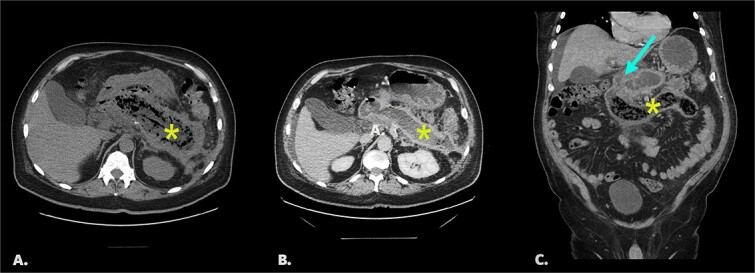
(A) Initial non-contrast CT. Axial image shows extensive pancreatic necrosis and phlegmon (*). 1B/C Follow up CT with IV contrast (portal venous phase). Image 1B: axial image shows fluid replacement of tissue in the pancreatic bed (*). Image IC: coronal image shows fistula between pancreatic bed and duodenum (arrow) and bezoar in the distal duodenum (*).
